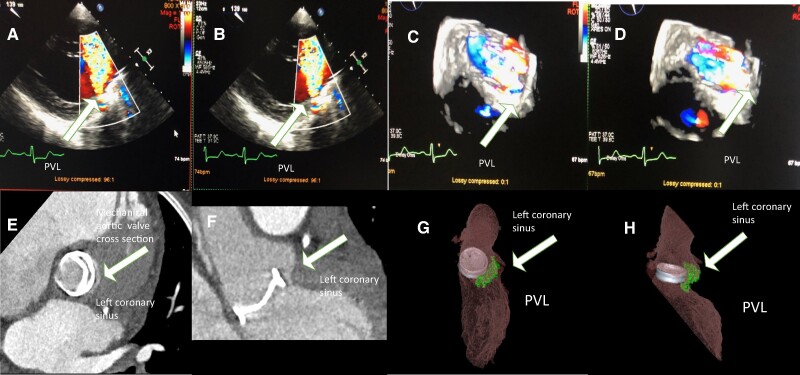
(*A*, *B*) Transoesophageal echocardiography images of a large-sized mechanical aortic valve. A white arrow points at the location in the left coronary sinus. (*C*, *D*) Three-dimensional TOE images of the location of the large-sized mechanical aortic valve paravalvular leak. The white arrows point at the large ostium of the leak. (*E*, *F*) Computed tomography angiography images of the mechanical aortic valve and the detected paravalvular leak. The white arrows point at the leak location. (*G*, *H*) A three-dimensional reconstruction of the mechanical surgical valve, paravalvular leak, and regurgitation volume. The white arrows point at the leak location in the left coronary sinus.
